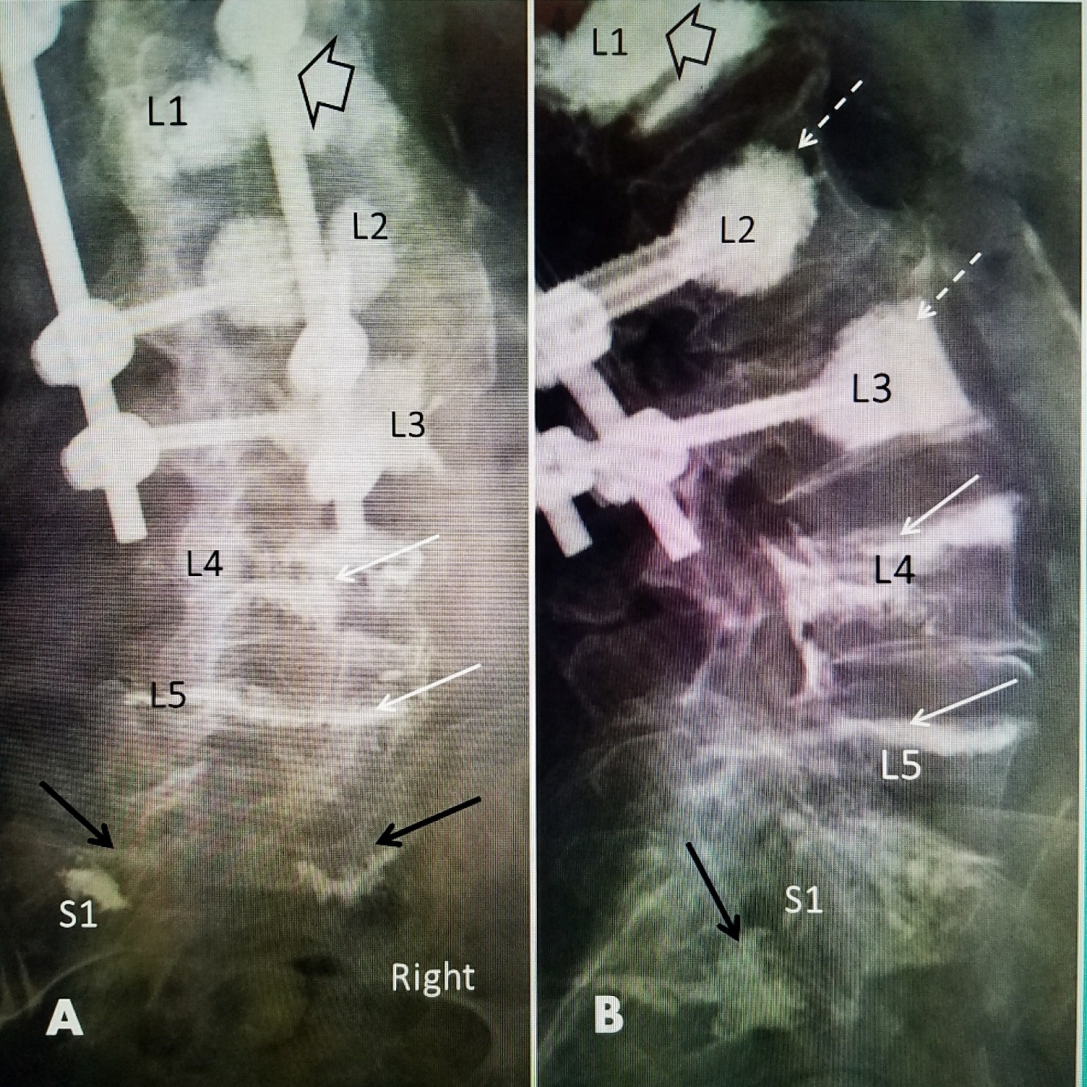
Post vertebroplasty films from patient in Figure 3 (a 78-year-old female) who had an L1 fracture and kyphoplasty followed two months later by T11 to L3 instrumentation. She went on to develop progressive cascading fractures: L4, L5, and sacrum A: Anteroposterior (AP) radiograph six months after original kyphoplasty at L1 showing 18^o^ dextroscoliosis. She had an L1 kyphoplasty (open, large black arrow) and then, two months later, T11 to L3 instrumentation with cemented bicortical screws (dashed white arrows). A bone scan showed mild uptake at L4, L5, and bilaterally in the sacrum and left ilium. Limited vertebroplasty at L4 and L5 can be seen (solid white arrows) and cement in the sacral alae (solid black arrow) B: Lateral radiograph showing that the L4 and L5 superior endplates were treated with limited preventive vertebroplasty (solid white arrows). The sacral fractures were also treated with superior placement of cement in the sacral alae (solid black arrow). Followup over 18 months did not show further progression of the L4 or L5 vertebral compression fractures.
